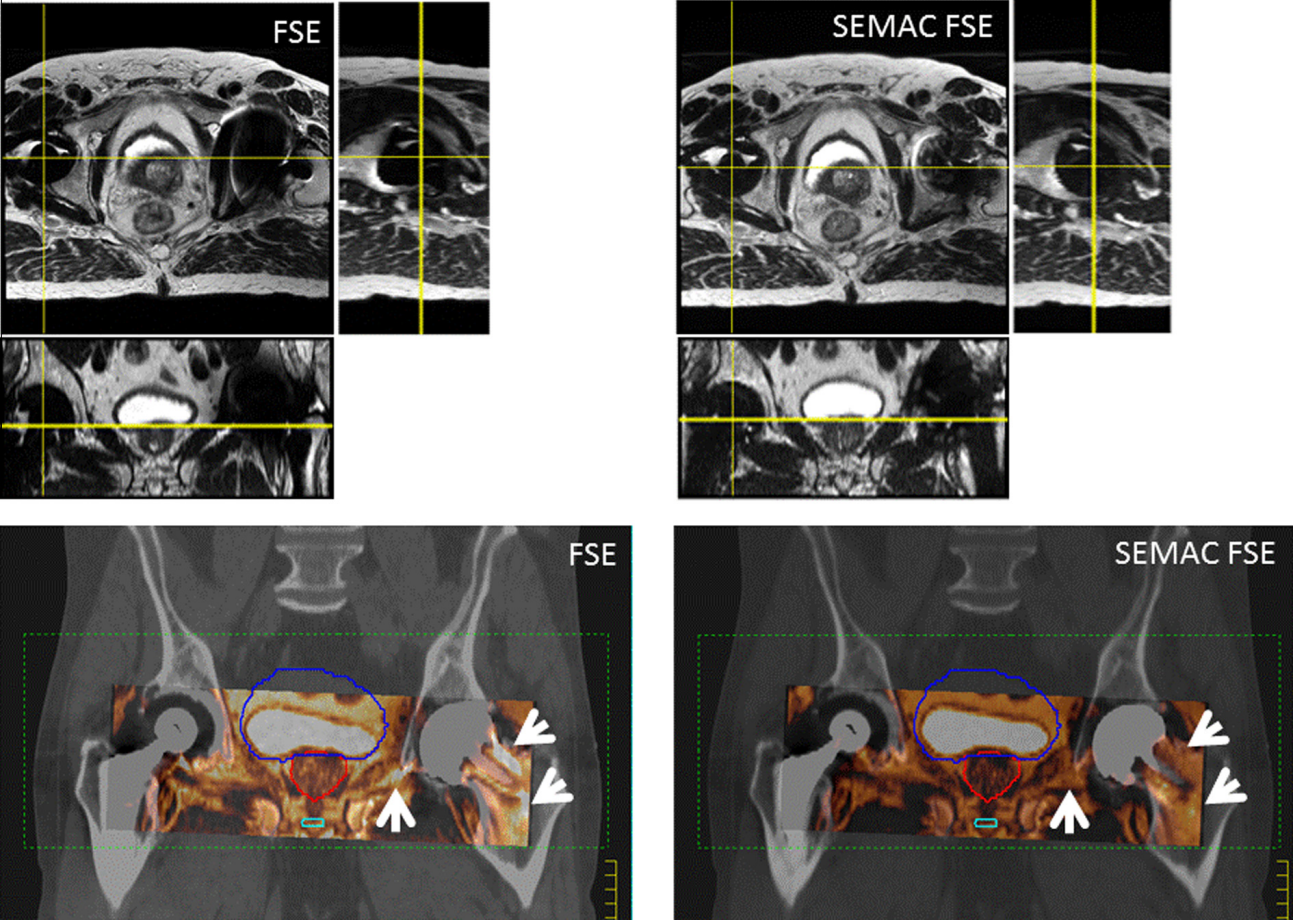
Conventional FSE (left) and SEMAC FSE (right) in patient with bi-lateral hip replacement (slice thickness 2.5 mm). The right hip (left in figure) is the least affected by signal loss, but geometrical distortion is still clearly visible in the conventional FSE, sagittal reconstruction. Patient position changed slightly between conventional FSE and SEMAC FSE acquisitions (bladder filling and rectal position). The registration with CT was undertaken by experienced personnel using all the information available. This task is hindered by the areas of signal pile up in conventional FSE images (arrows). Signal loss and signal pile up are reduced in SEMAC FSE images.
